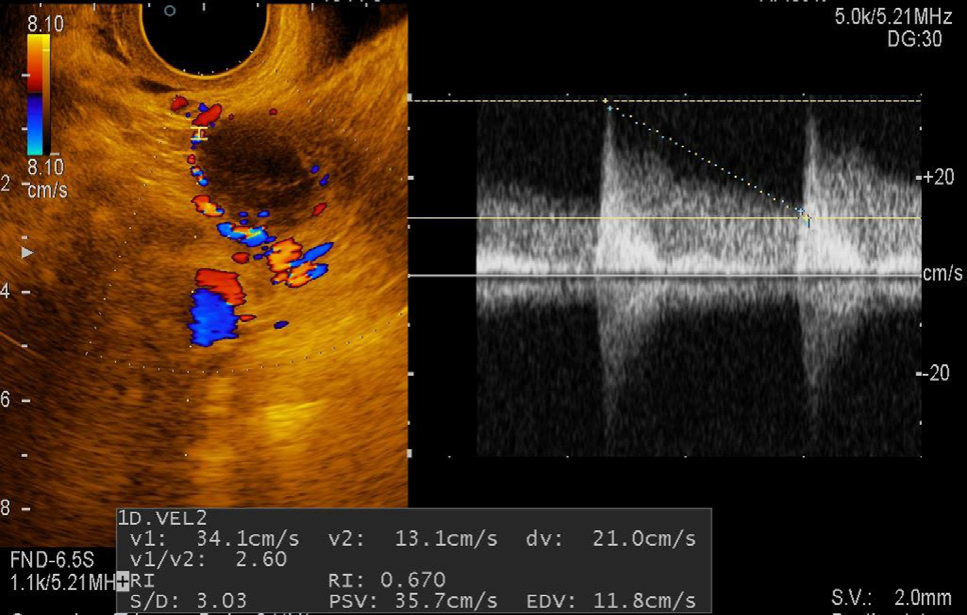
Sonogram of an endometrioid cyst with Doppler ultrasonography.
